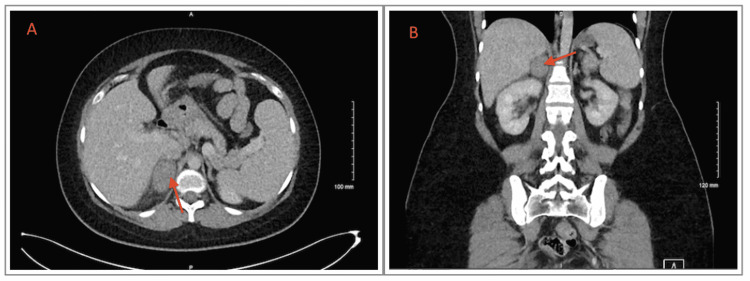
Axial (A) and coronal (B) views of CT scan demonstrate a right heterogeneous adrenal mass (arrows) consistent with hemorrhage.
